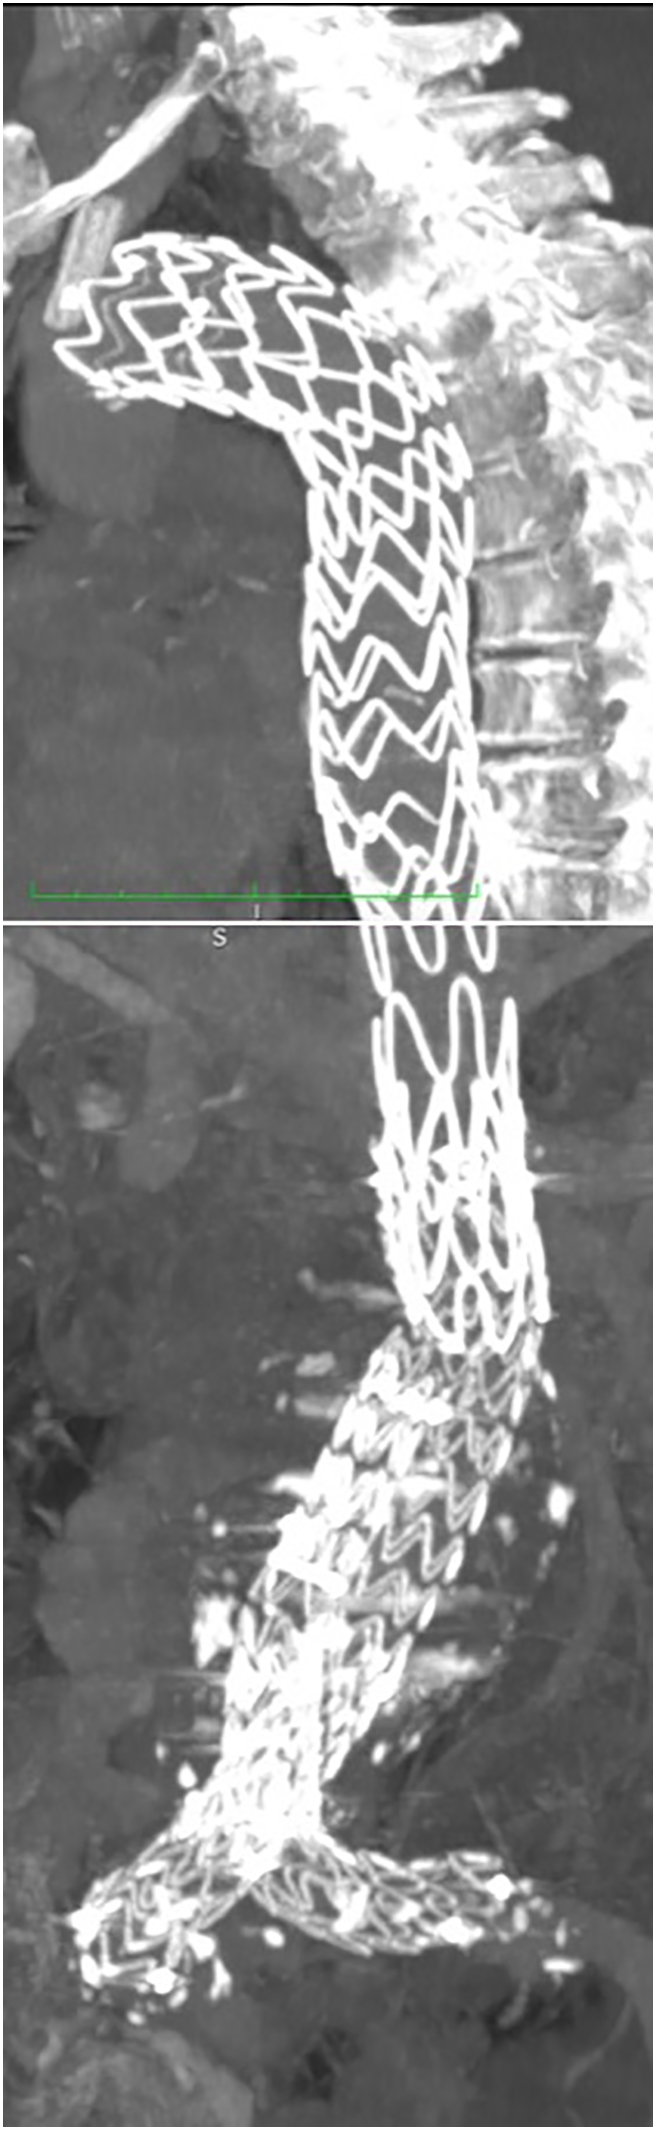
Post-operative CT MPR after the first procedure showing Thoracic graft (A) and abdominal graft (B).
